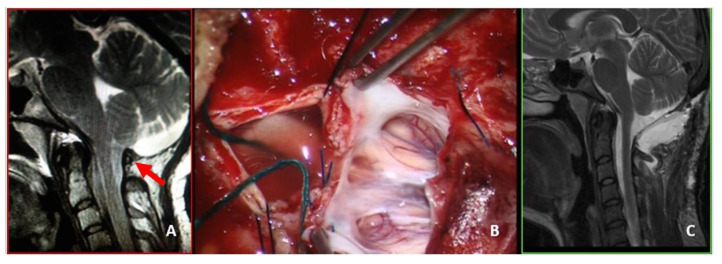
This 29-year-old woman had undergone osteodural decompression without laminectomy. One year later, she was admitted to our department with an untreatable persistent hiccup responsible for difficult alimentation, invalidating headache, dizziness, tinnitus, fever, rigor nucalis, and diffuse asthenia. An infection was suspected, but was ruled out. (A) Preoperative MRI, T2-weight, sagittal view showing a relatively wide craniectomy with C1 (red arrow) still compressing the arachnoid space, and a scarce presence of CSF around the CM-1. She underwent laminectomy and dural sac augmentation. (B) Intraoperative picture depicting a diffuse, tight adherence between the dural graft and the parenchyma. To replace the graft, a meticulous dissection was necessary. Finally, the dural sac was repaired with a synthetic graft. (C) Postoperative MRI, T2-weight, sagittal view showing a well-bathed CM-1. An asymptomatic pseudomeningocele was detected. This remained completely asymptomatic, even though it was still evident (but reduced) 6 months later. One year after the reoperation, the patient reported sporadic headache bouts, but had no other symptoms. The final CCOS score was 13.
